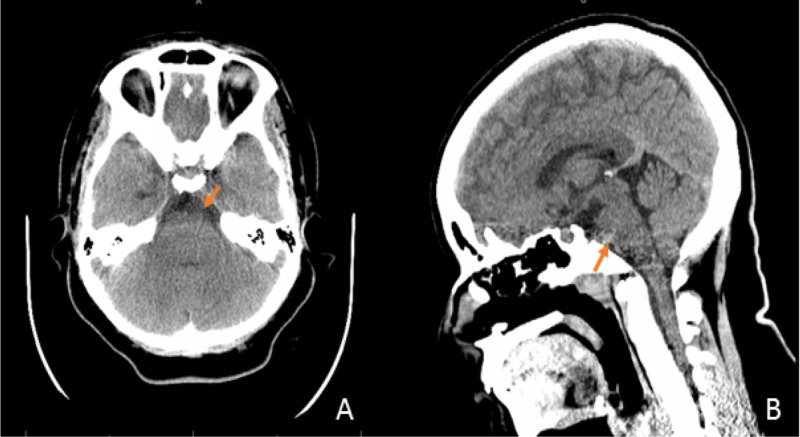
CT head without contrast at admission CT head without contrast performed at admission: an isodense retroclival mass with mild mass effect on the pons (arrow). A: axial view; B: sagittal view. This was read as unremarkable before the brain MRI was performed CT: computed tomography; MRI: magnetic resonance imaging
